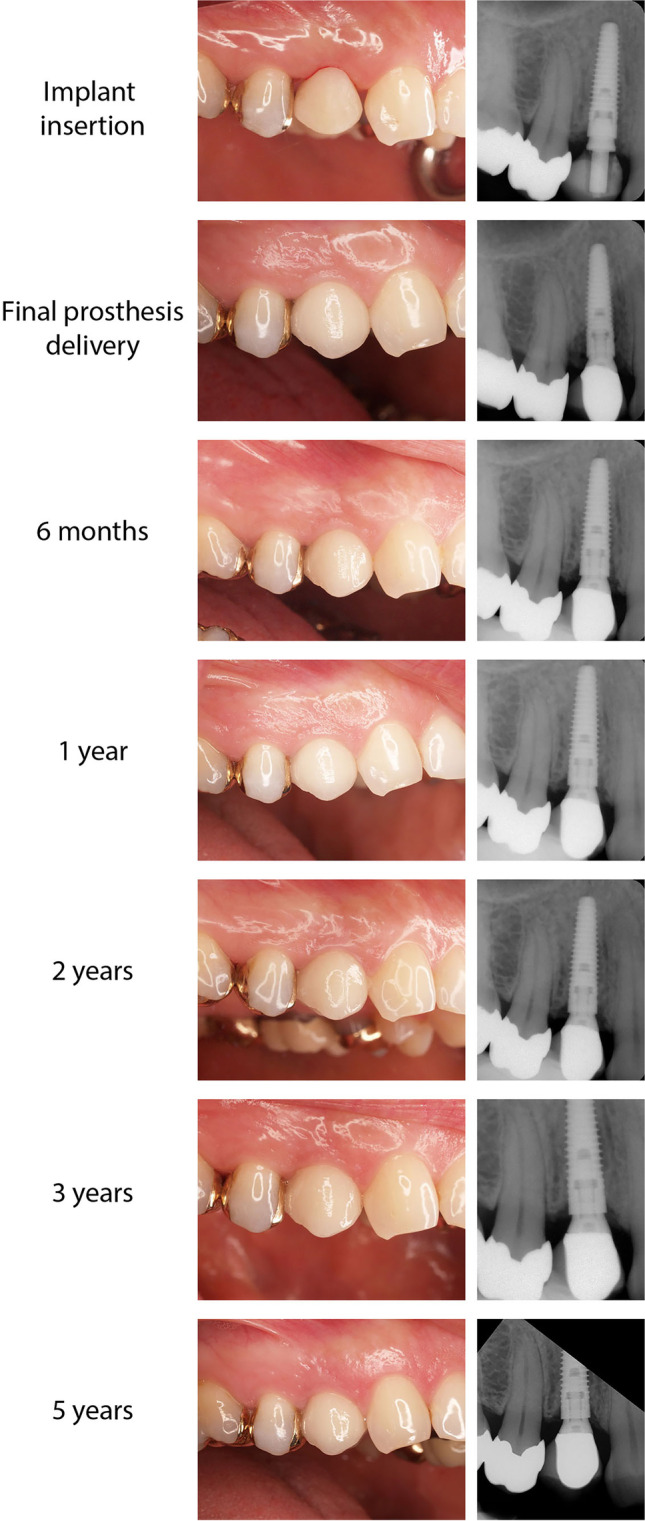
Representative clinical case. A 45-year-old female (nonsmoker) with no history of periodontitis or other parafunctional tendencies received a 13-mm long and 3.5-mm diameter NobelReplace CC implant to replace the missing first premolar tooth in the maxilla (FDI position 14). The bone at the insertion site was assessed as hard (quality 2), and the final insertion torque was 45 Ncm. The implant was immediately provisionalized, and the patient attended follow-up visits until study completion. Clinical images (left panels) and radiographs (right panels) acquired at indicated time points reveal healthy hard and soft tissue responses, accompanied by a visible improvement in esthetics
